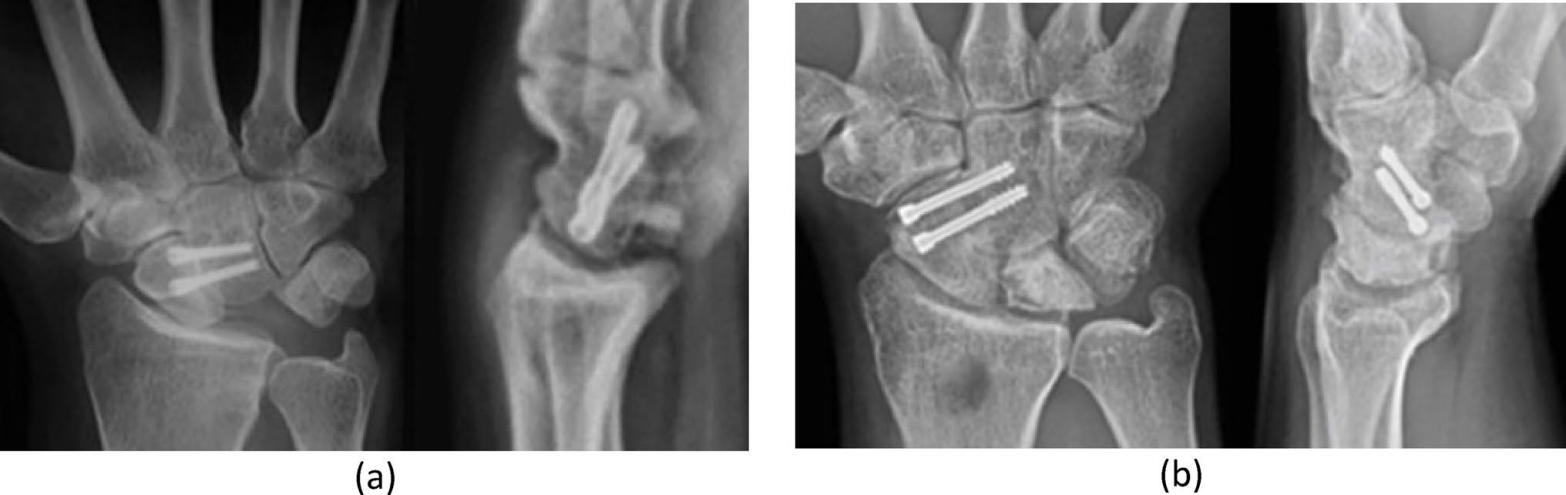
Postoperative radiographs after SC arthrodesis with lunate excision. Anteroposterior and lateral views showing scaphocapitate fusion and removal of the necrotic lunate Postoperative radiographs after SC arthrodesis with lunate preservation. Anteroposterior and lateral views showing preserved lunate
